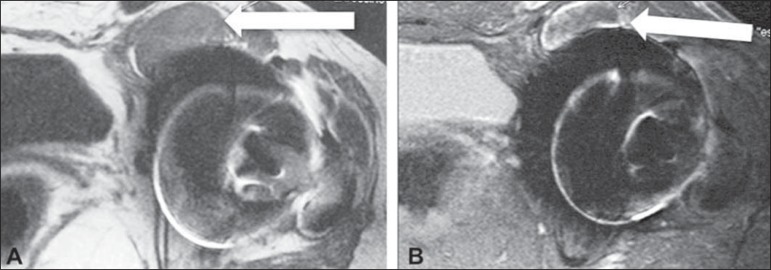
A: Axial, T1-weighted section demonstrating anterior collections
